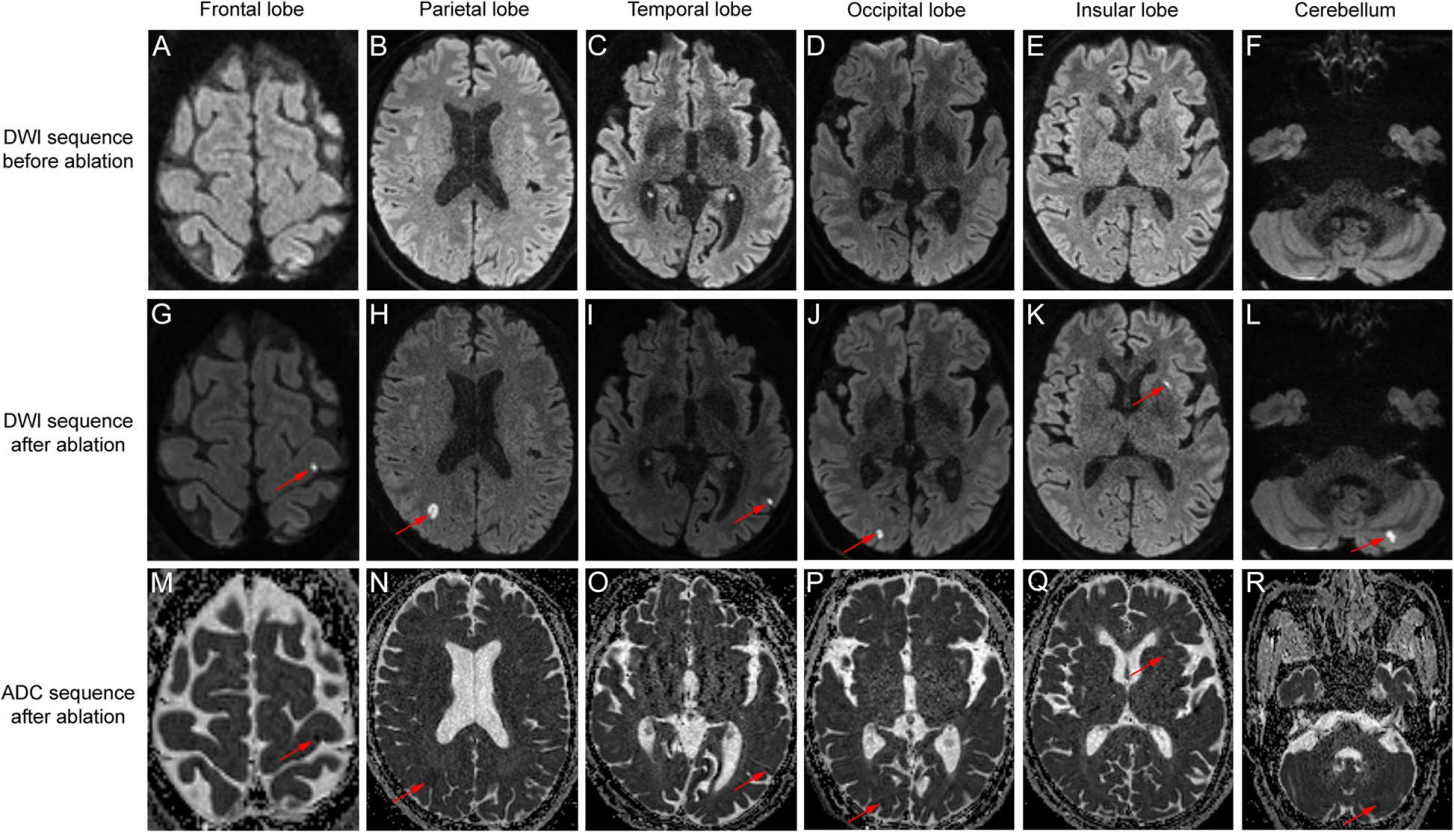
The representative acute lesions identified by high-resolution diffusion-weighted magnetic resonance imaging. DWI Diffusion-weighted magnetic resonance imaging, ADC apparent diffusion coefficient magnetic resonance imaging
